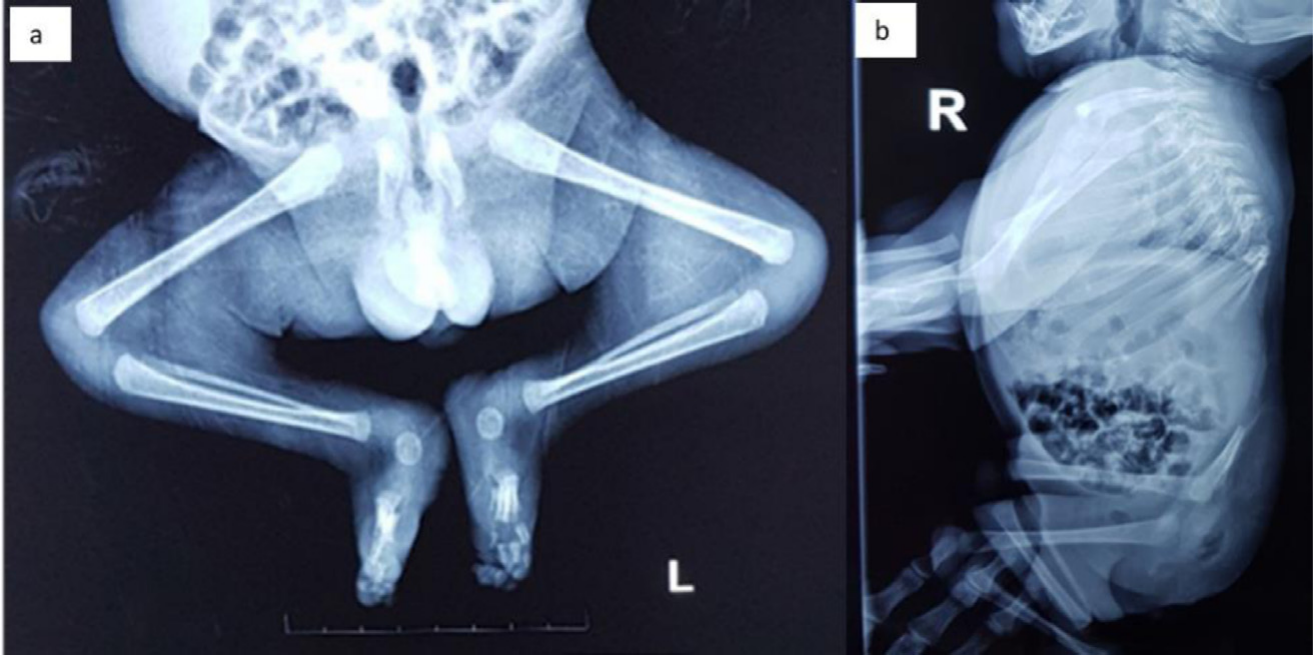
(A) Radiography of whole spine and lower extremities showed hypoplastic ilium and ischium bones. The flexion position was due to the stiffness in the lower extremities, with bilateral clubfoot deformity. (B) In lateral view, signs of partial sacral agenesis and underdevelopment of the lower thoracic, lumbar spine, and sacrum were visible.
